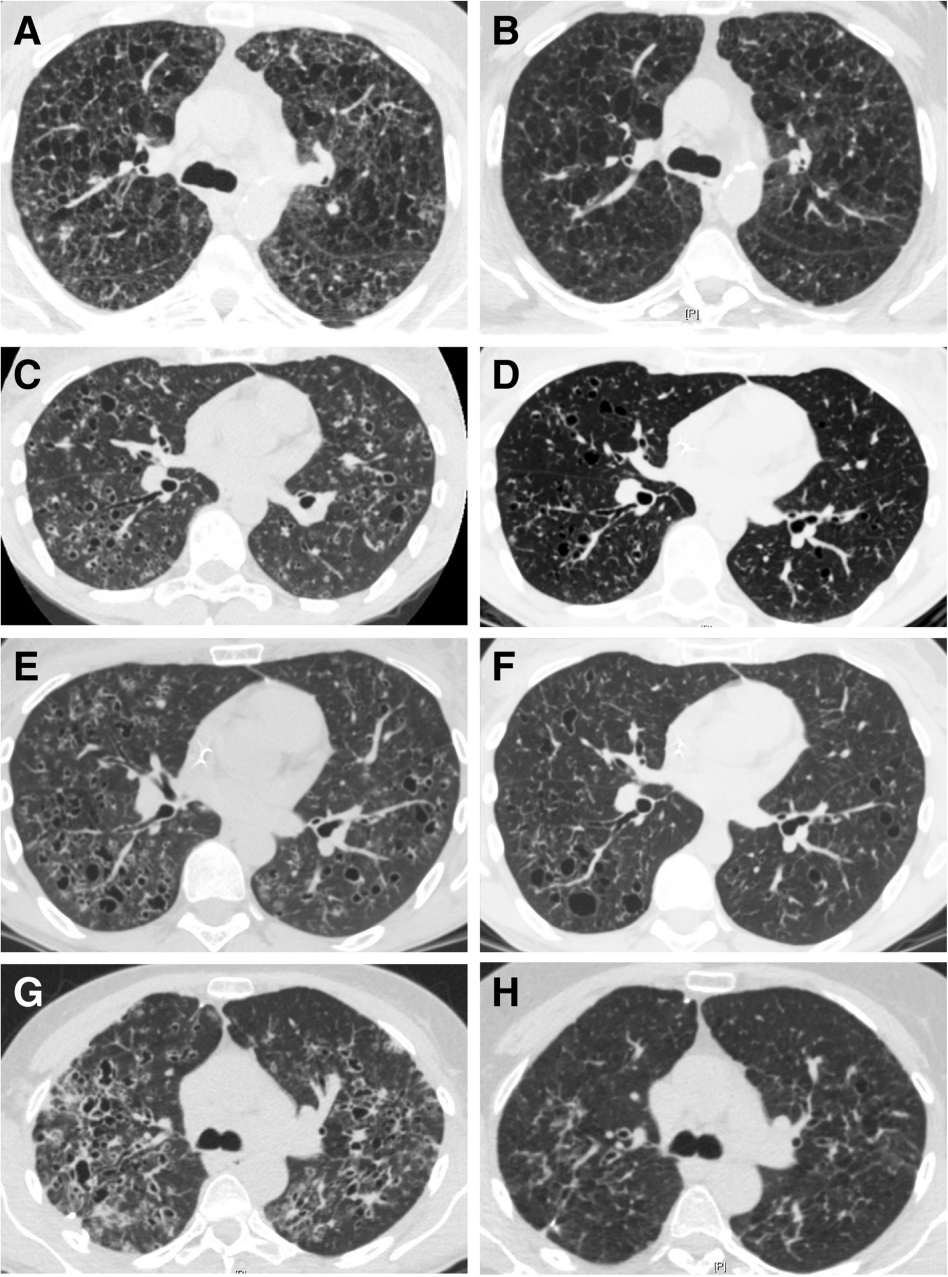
Representative HRCT features before (A, C, E, G, I, K) and after cladribine therapy (B, D, F, H, J, L) in 3 patients. A, B: patient 1; C, D: patient 2; E, F: patient 2 (relapse); G, H: patient 3.
